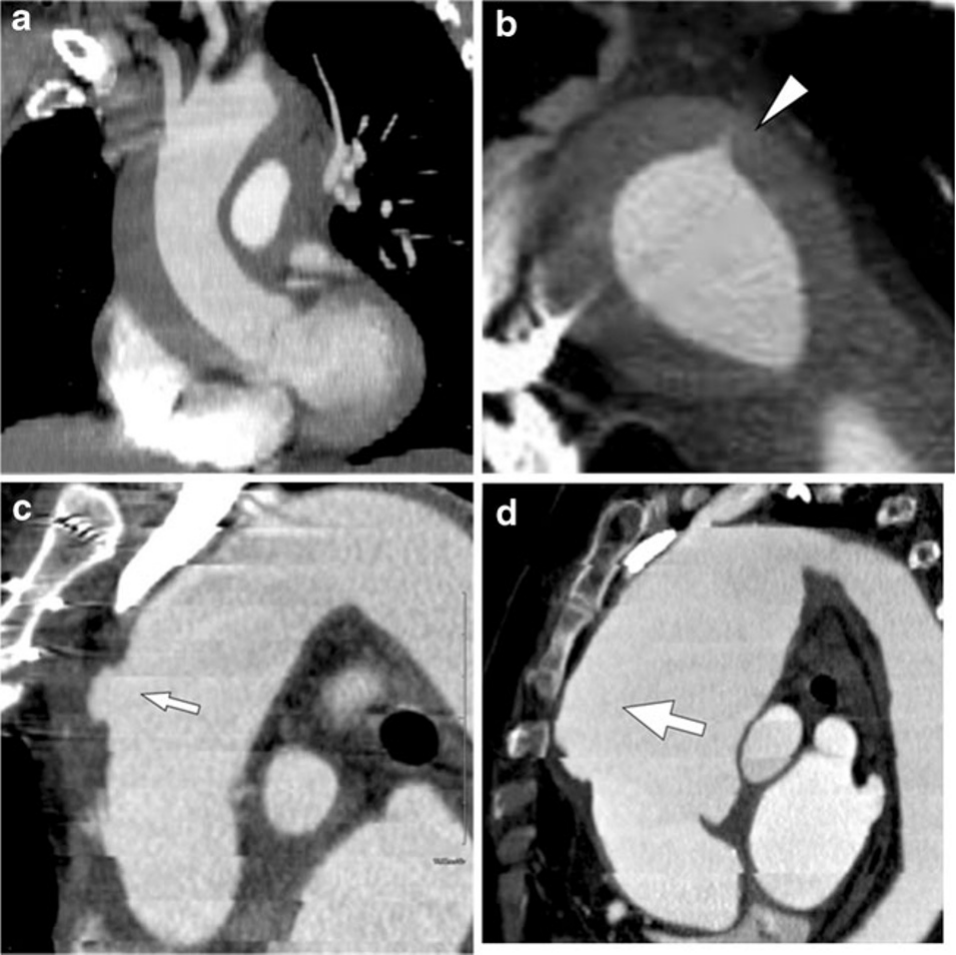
Aneurysmal dilatation of the aorta secondary to dissection variant IMH in a 66-year-old woman. a Oblique sagittal contrast-enhanced CT demonstrates IMH extending from the ascending aorta through the proximal descending aorta. b Axial image depicts a streak-form ulcer-like projection (primary intimal tear) in the descending aorta (*arrowhead*). c Two days after onset, an ulcer-like projection was enlarged to form an outpouching cavity into the thrombosed false lumen (*small arrow*). d One week later, the haemorrhagic content is resolved and the ulcer-like projection not been detected. On the other hand, the aorta shows aneurysmal dilatation. Note that the dilatation starts from the location where the haematoma was present (*large arrow*). Although the pathological features and clinical course are different, such aneurysmal dilatation of dissection variant IMH might be confused with an atherosclerotic aortic aneurysm if an ulcer-like projection is not detected on the initial CT examination
